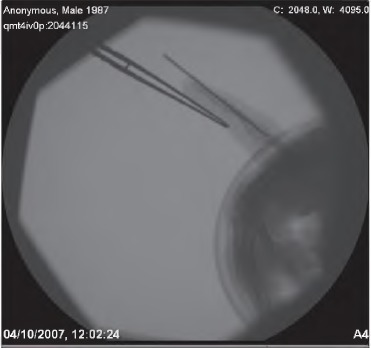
Lateral x-ray of finger with radiolucent kidney dish
